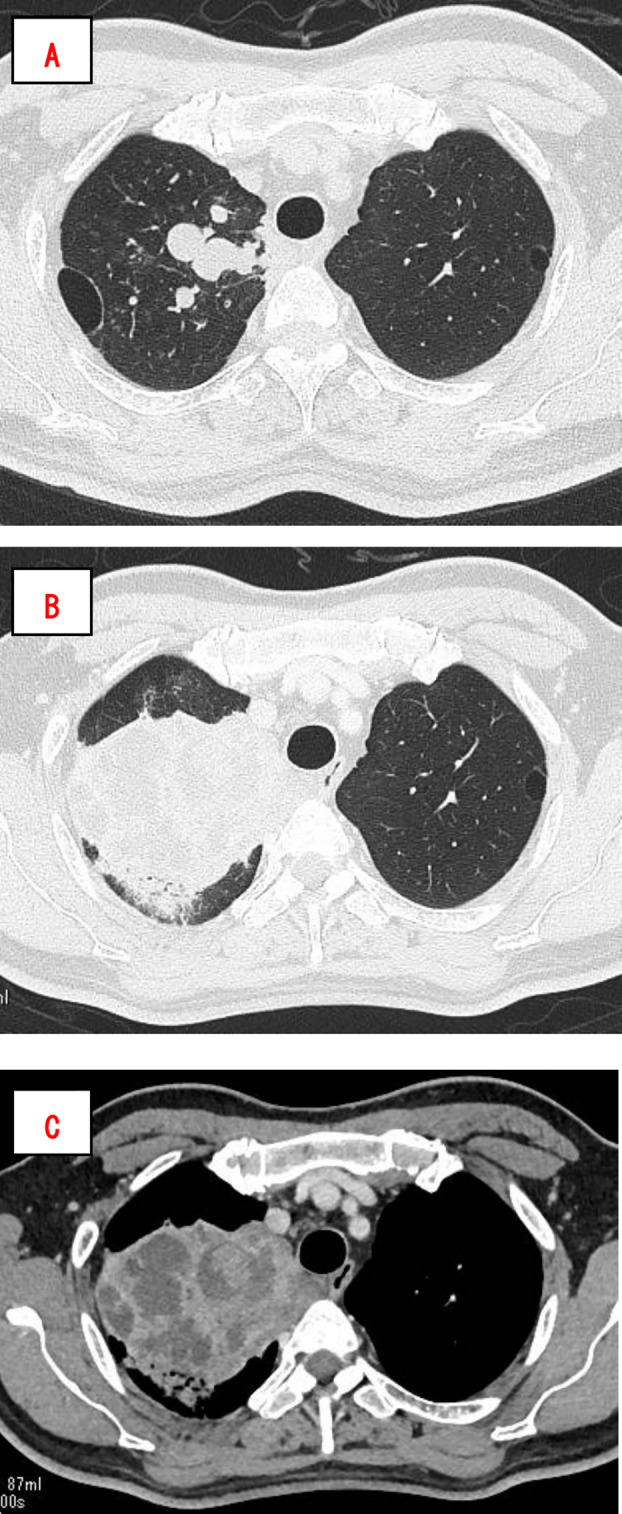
Imaging findings. CT showed a lobulated mass in the right upper lobe, with a maximum diameter of 41 mm (A). CT performed 1 month after the initial CT scan revealed that the tumour was filled with soft tissue density areas and had enlarged, accompanied by peripheral consolidation suggestive of obstructive pneumonia (B, C). CT, computed tomography.
